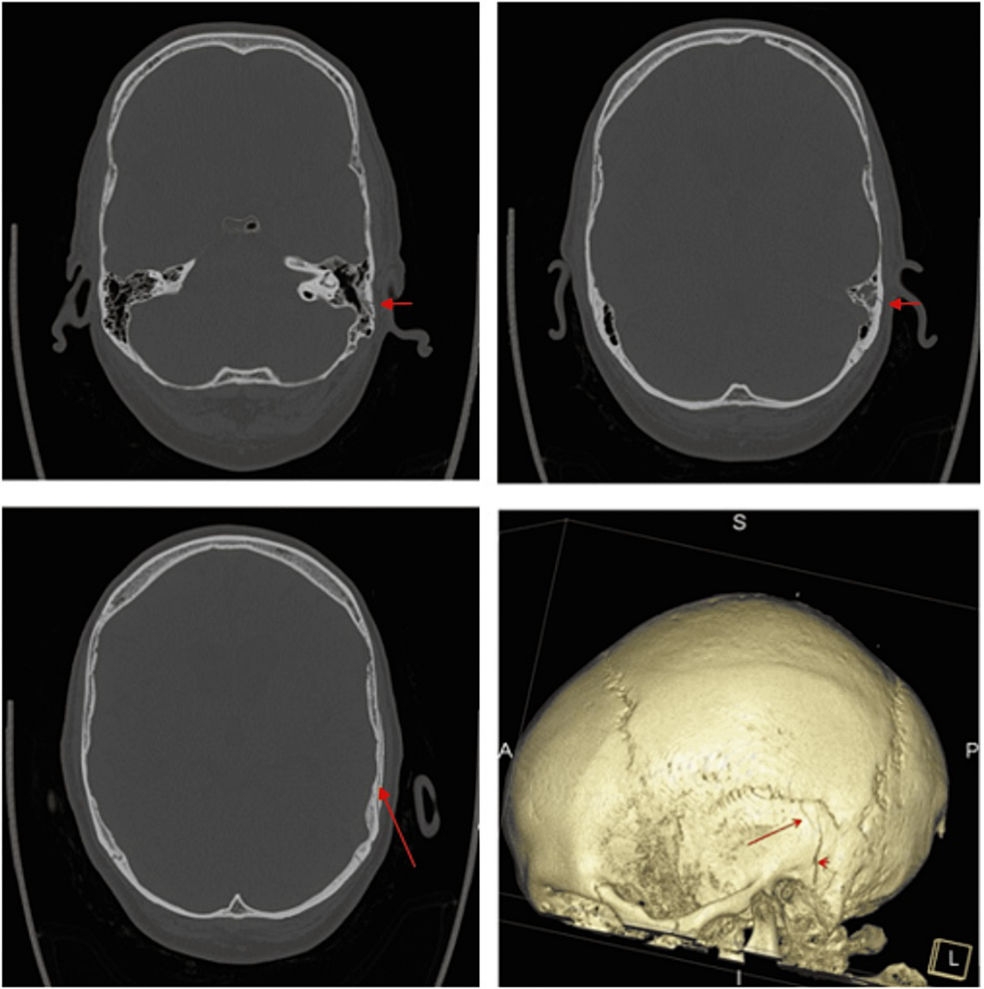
CT head showing undisplaced left mastoid and longitudinal squamous temporal fracture.
